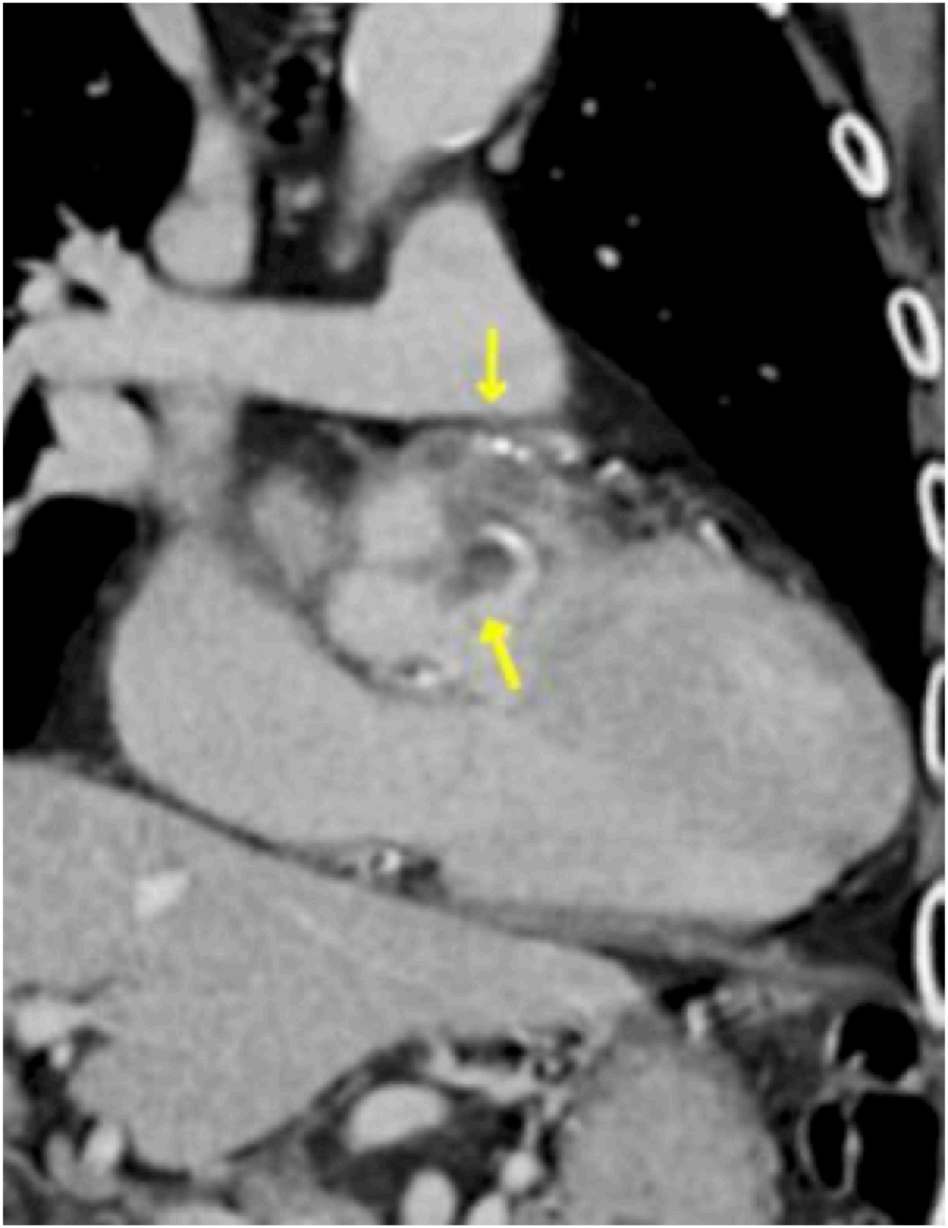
Chest computed tomography (CT) scan with contrast performed upon admission showing an area with poor contrast enhancement on the cranial side of the aortic root (yellow arrows), suggestive of an abscess.
